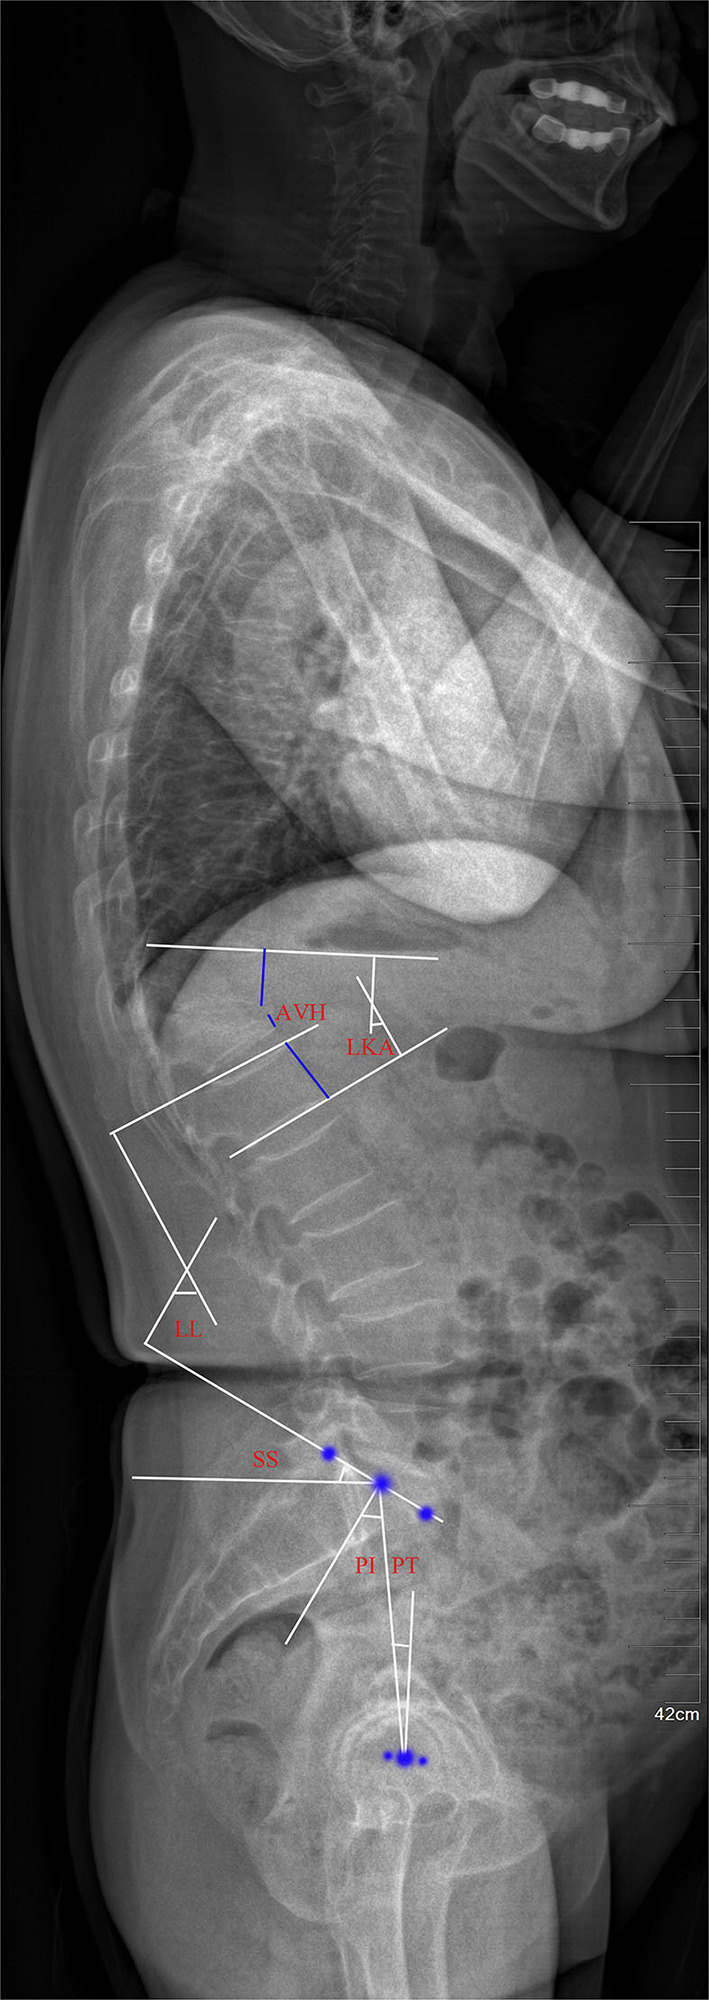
Plain lateral radiograph for measuring radiographic parameters. AVH, anterior vertebral height; LKA, local kyphotic angle; LL, lumbar lordosis; SS, sacral slope; PI, pelvic incidence; PT, pelvic tilt.
